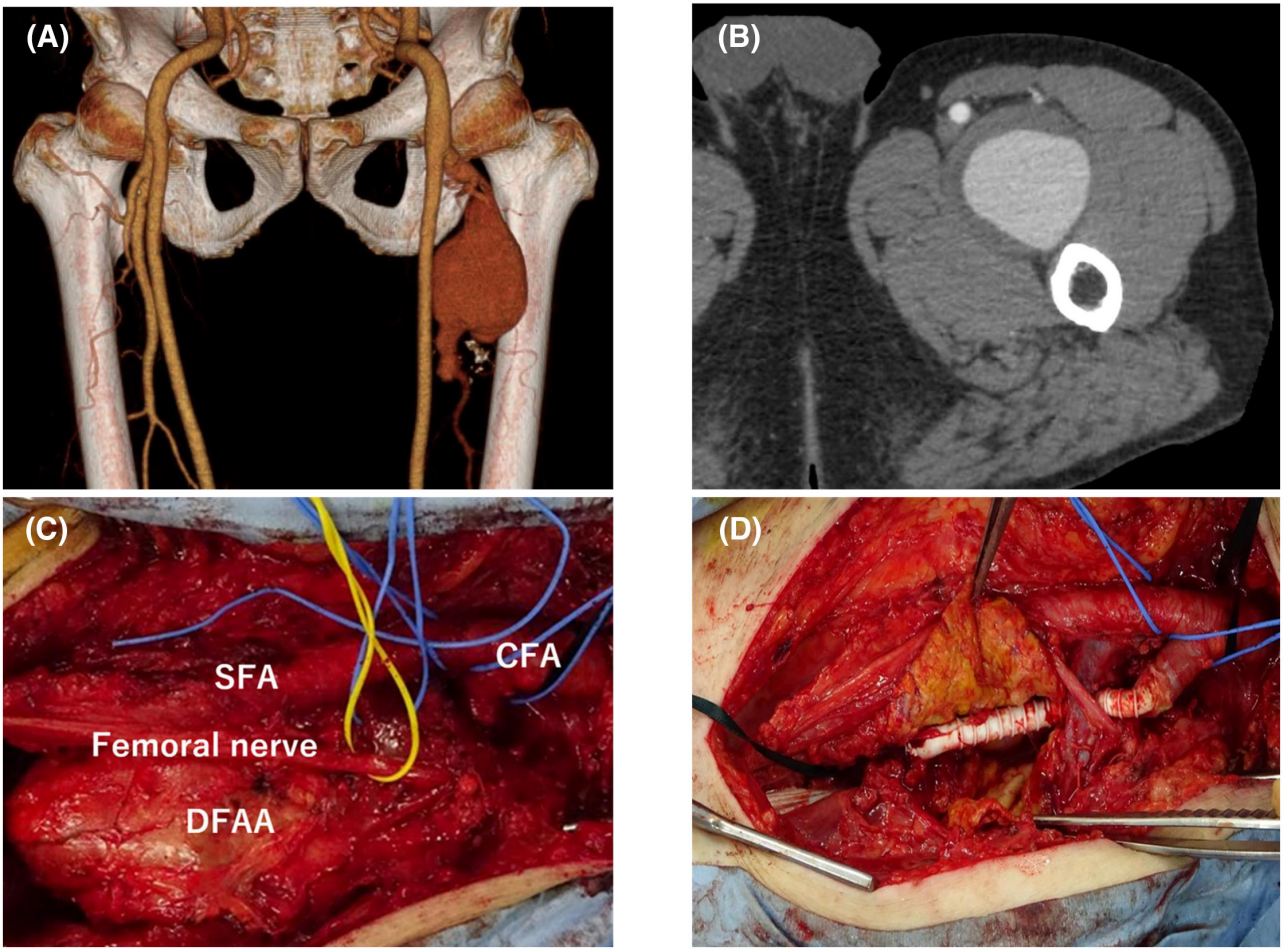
Computed tomography (CT) showed huge left DFAA (A) and abundant thrombus in the sac (B). Femoral nerve was carefully dissected from DFAA (C), and graft replacement was performed (D). DFAA, deep femoral artery aneurysm.
